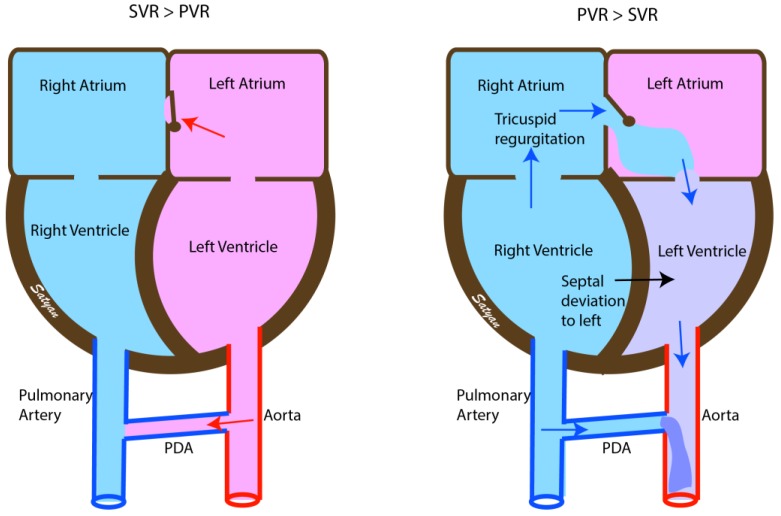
Echocardiographic findings in normal infants (left) and in PPHN (right). Soon after birth the pressures within the left-sided chambers of the heart are higher than in the right and the fetal shunts are reversed. The interatrial shunt and the shunt across the patent ductus arteriosus (PDA) is left to right. In infants with PPHN the pressures remain elevated in the right atrium and ventricle with right to left shunt at the atrial level and at the PDA causing desaturation (due to interatrial shunt) and differential cyanosis (due to PDA). There is right ventricular hypertrophy with bulging of the interventricular septum to the left and tricuspid regurgitation. SVR—Systemic vascular resistance, PVR—Pulmonary vascular resistance. Copyright Satyan Lakshminrusimha.
